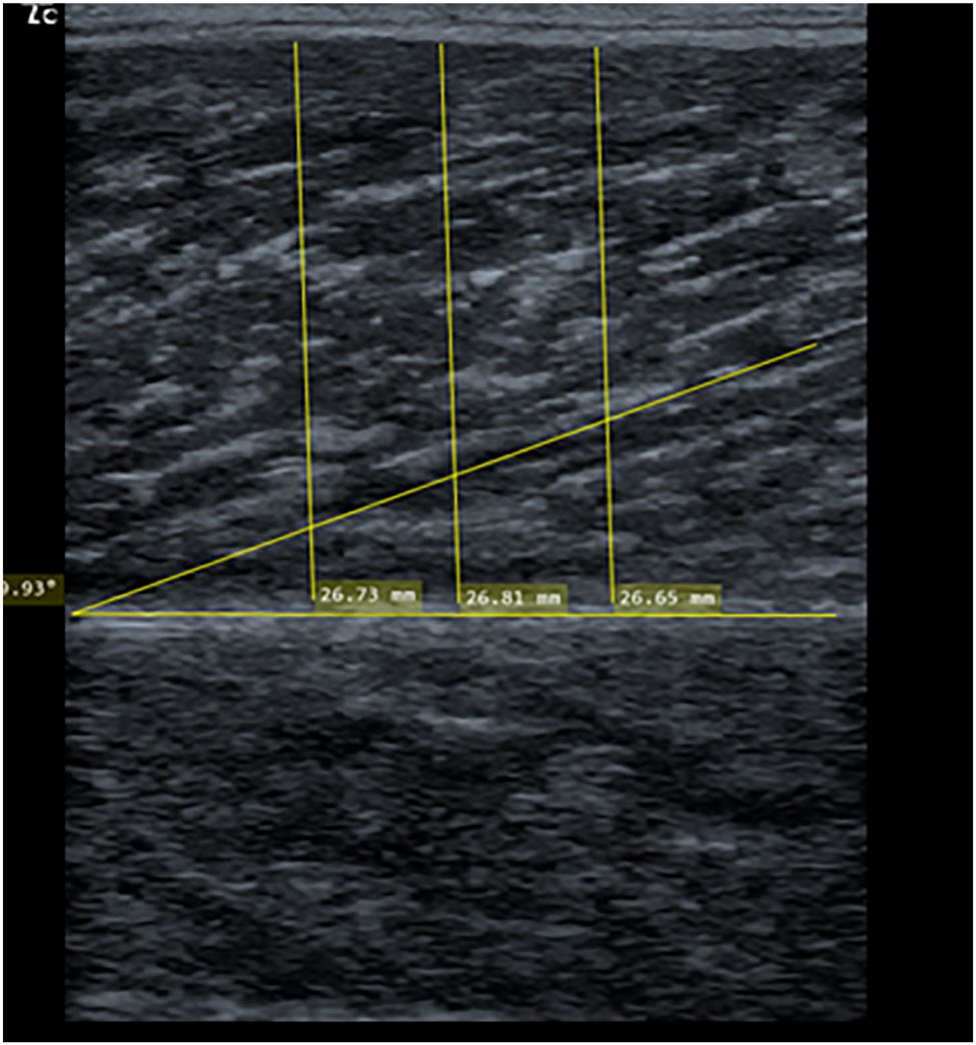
Sonography to investigate muscle thickness and pennation angle in the calf muscle
